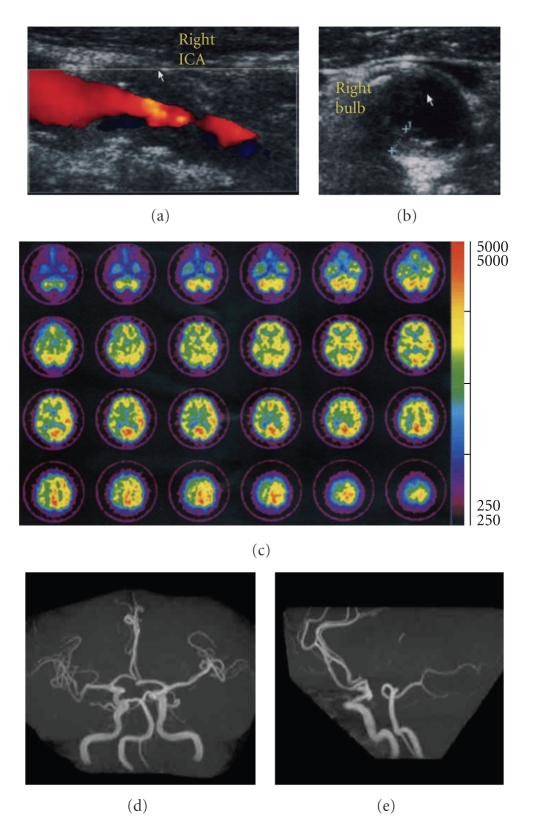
(a) B-mode ultrasound with color flow Doppler image on the longitudinal display of the carotid plaque with irregular surface. (b) The transverse display of the plaque at the carotid bifurcation. (c) ^123^I-IMP SPECT transaxial slices of a patient with right ICA stenosis. MR angiography ((d) submental vertical projection; (e) lateral projection) with a hypoplastic or absent anterior and posterior communicating arteries.
